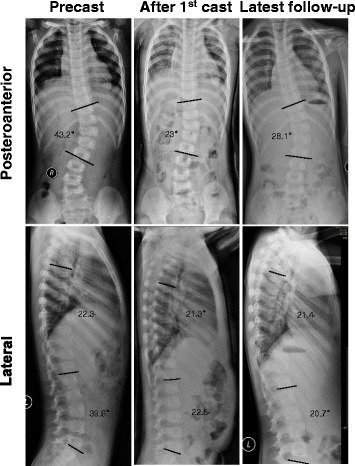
Radiographs of a representative case of non-CS patient (case no. 15). A 13-month-old female patient visited our clinic due to scoliosis. X-ray examination revealed IIS. The patient was unable to receive bracing treatment due to her young age, and the scoliosis continued progress. Therefore, the patient started receiving casting treatment at 2.75 years old. The scoliosis became stable after eight times of casting correction, and then, the patient wore a brace
